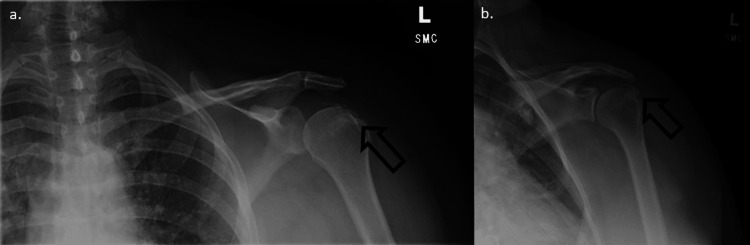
Radiographs of the left shoulder demonstrating rotator cuff calcific tendinitis. a) Anterior-posterior view. b) Internal rotation view.
